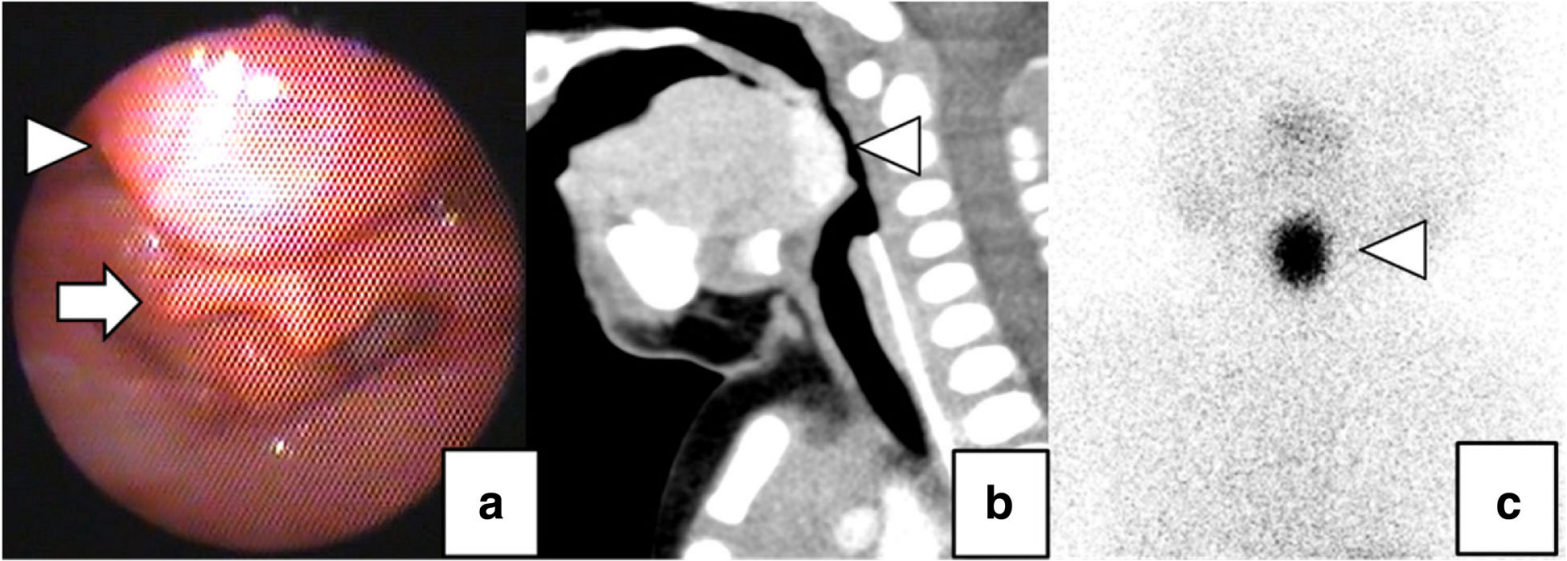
Preoperative imaging tests. Laryngeal endoscopy revealed a lingual mass (arrow head) compressing the epiglottis (arrow) (a). Enhanced CT (b) and thyroid scintigraphy using iodine-123 (c) revealed that the mass (arrow heads) was an ectopic thyroid and that there was no normal pretracheal thyroid gland
